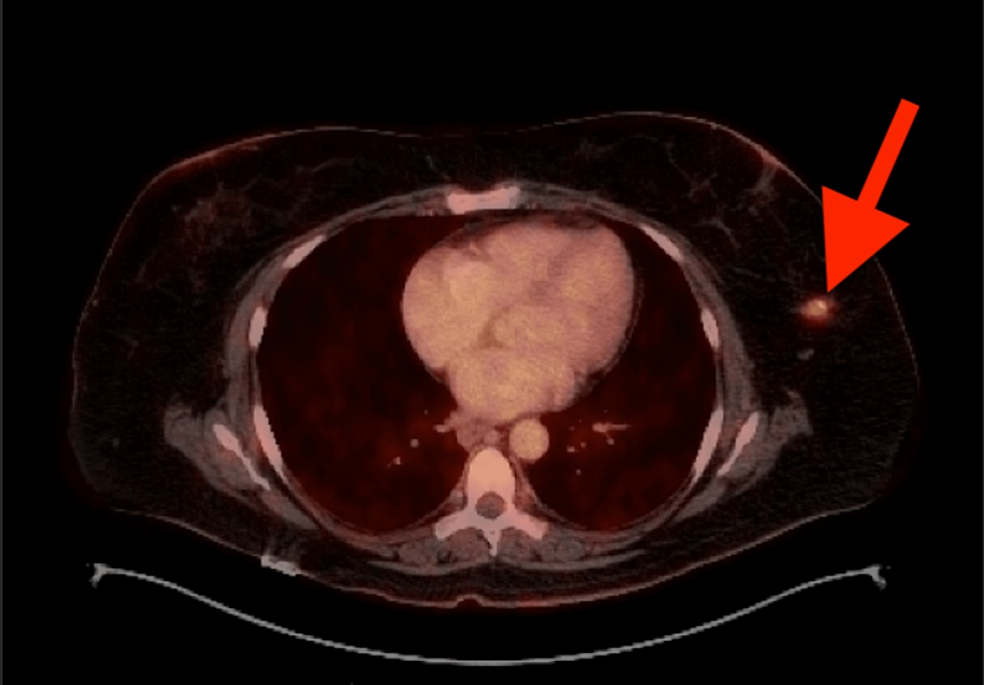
PET scan demonstrating a solitary FDG avid lesion in the left breast, measuring 1.6 cm in diameter. There was no evidence of regional adenopathy or distant metastatic disease. PET, positron emission tomography; FDG, fluorodeoxyglucose
